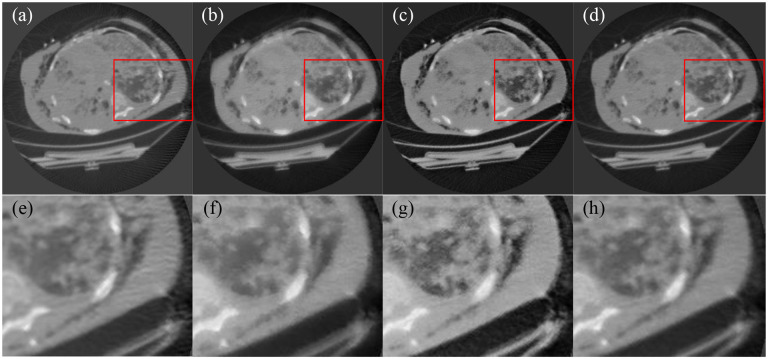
Comparison of the CT image reconstructions from GE scanner raw data of 246 projection views. (a) The image reconstructed using FBP, (b) the image reconstructed by SART with total variation, (c) the image reconstructed by score function-based method, (d) the image reconstructed by MSF reconstruction method, and (e–h) the enlarged image in the red rectangle in images (a–d), respectively.
